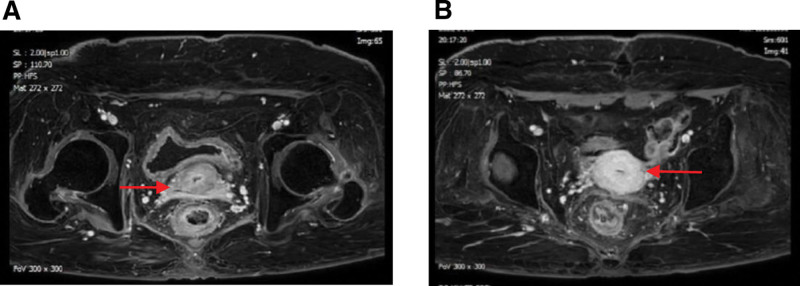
Pelvic contrast-enhanced MRI, showing the disappearance of the inhomogeneous enhancement mass (arrow) in the right part of the cervix(A) and the posterior part of the uterine body(B), the uterus basically return to the normal shape, and the bladder and rectal wall are thickened (after radiotherapy for CC). CC = cervical cancer, MRI = magnetic resonance imaging.
